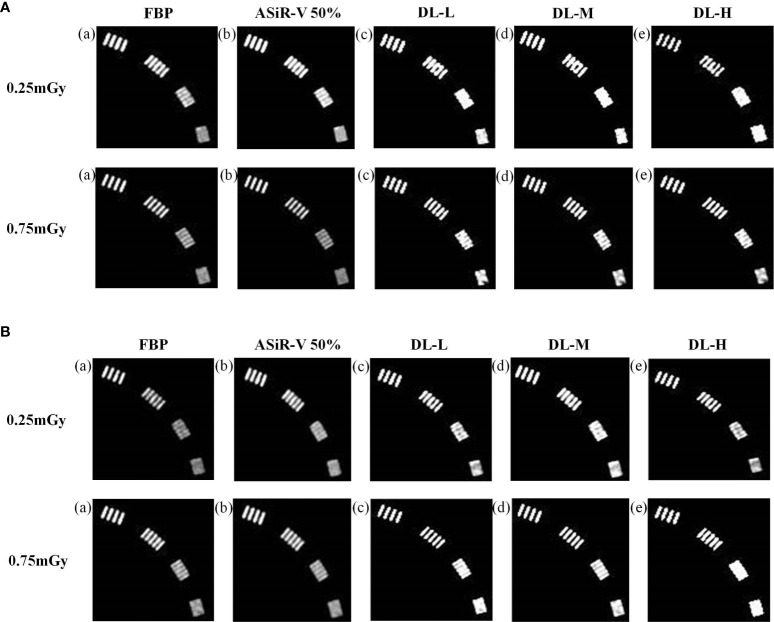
High-contrast images in axial mode reconstructed with FBP (a, f), ASiR-50% (b, g), and DLIR at three levels (L/M/H) (c, h; d, i; e, j) at 0.25mGy and 0.75mGy with a slice thickness of 1.25mm (A) and 5mm (B), respectively. CT, computed tomography; DLIR(L/M/H), deep-learning image reconstruction, level low, medium, and high; mGy, milligray; ASiR-50%, adaptive statistical iterative reconstruction-veo 50%.
